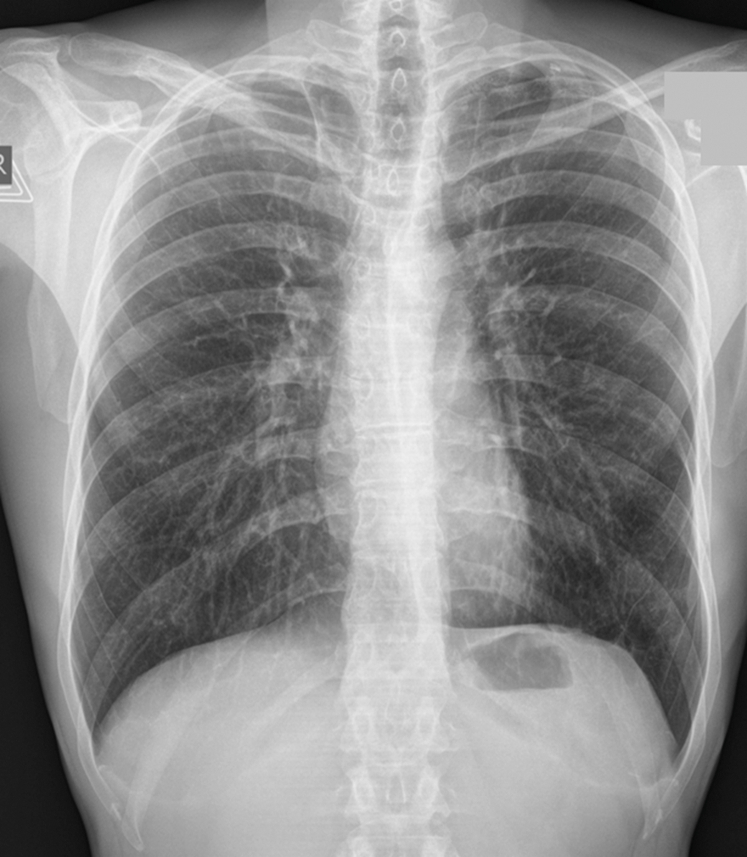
Follow-up chest radiograph on referral to our center. Complete resolution of the prior pneumothorax, with full reexpansion of the left lung.
